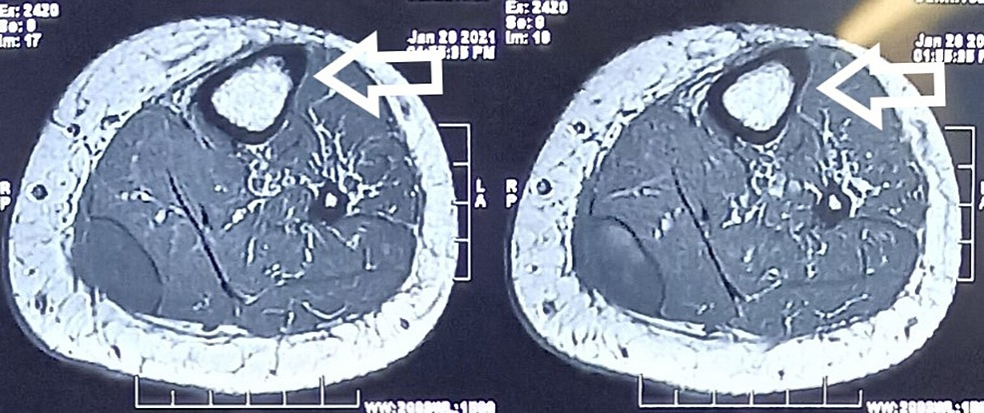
Axial T1-weighted image showing hypointense cyst (arrows)
